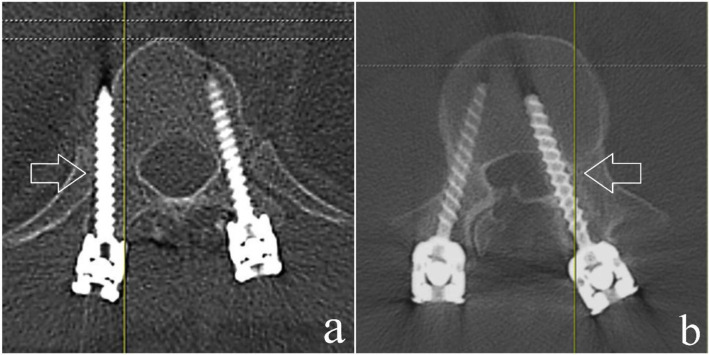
Post-operative axial CT image depicting a) Minor breach (<3 mm) and b) Major breach (≥3 mm).
